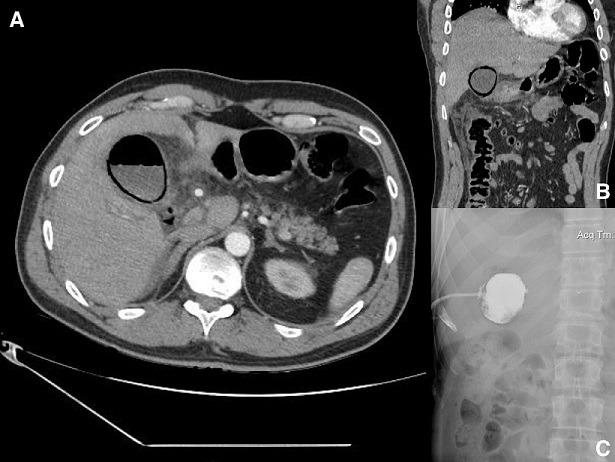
A) CT scan, axial, air within the gallbladder wall and lumen; B) CT scan, coronal, gaseous halo in the gallbladder wall; C) percutaneous cholecystostomy, complete obstruction of the cystic duct
